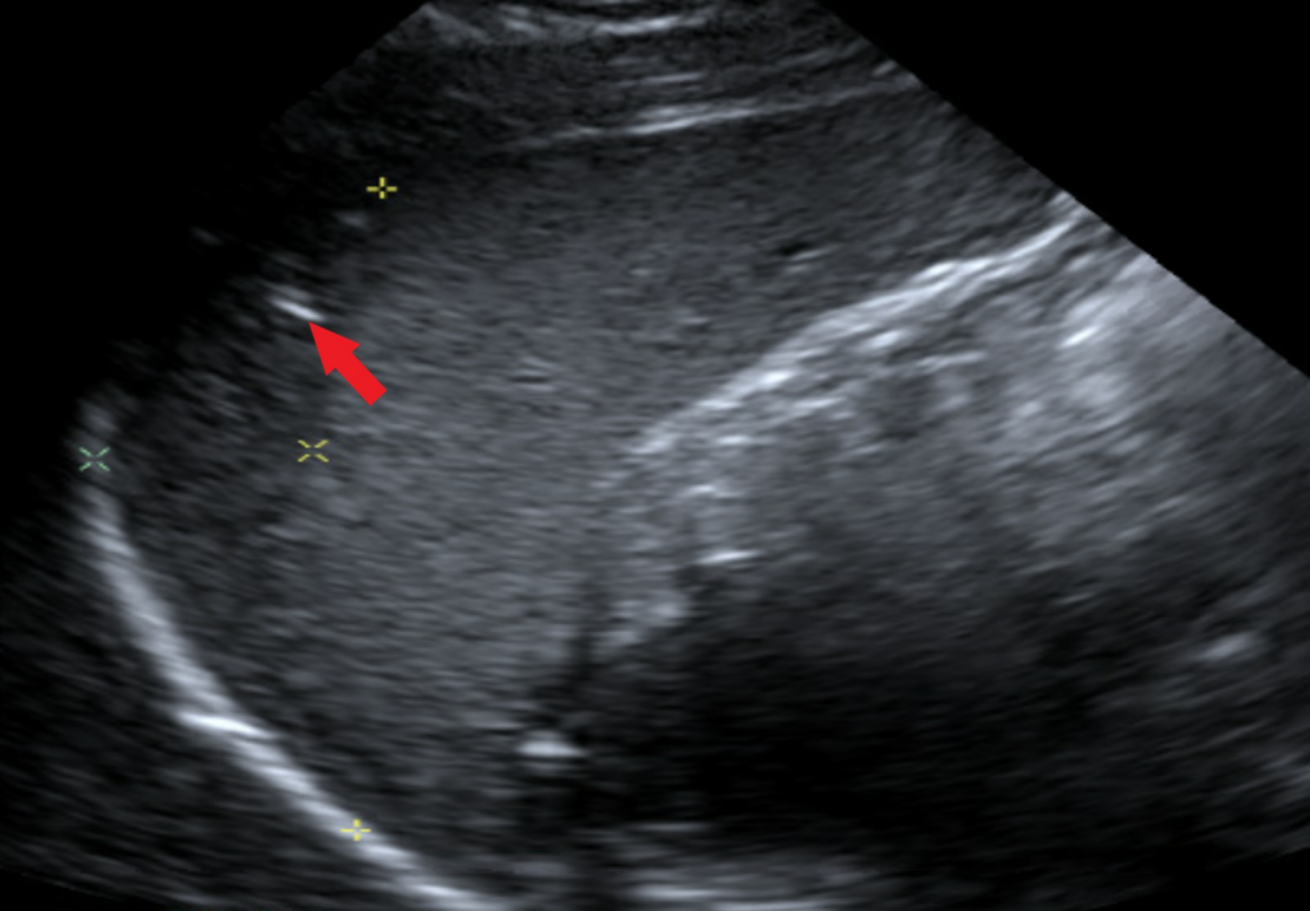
Complete abdominal ultrasound (US): Crescentic hypoechoic material around the edge of the spleen, underlying the dome of the left hemidiaphragm.
